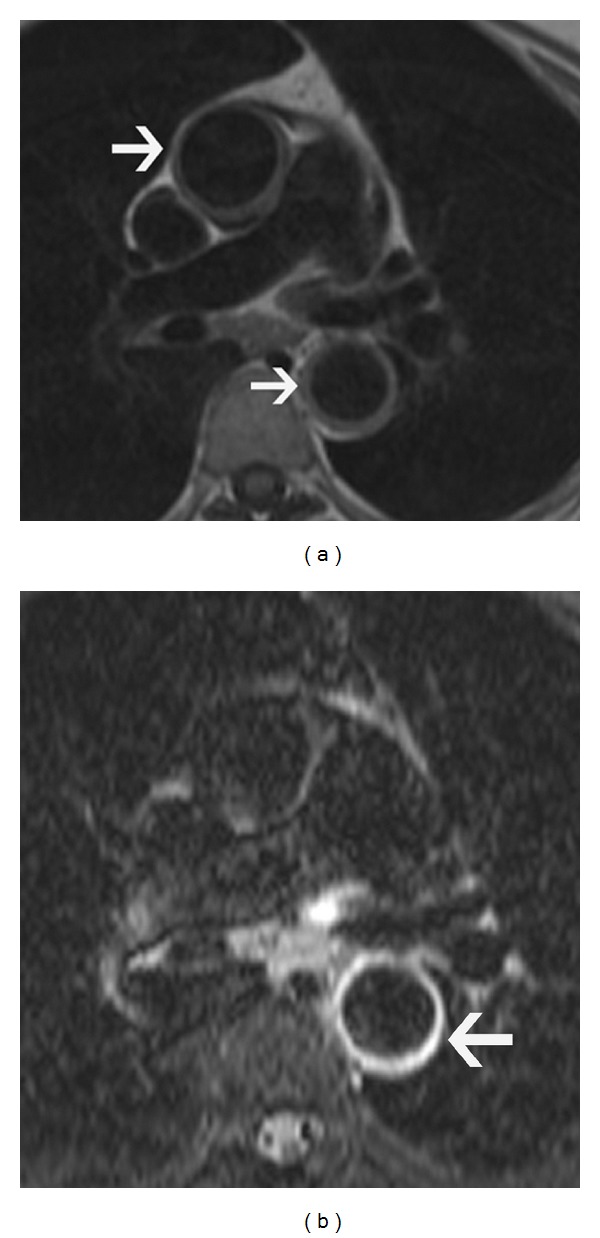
Giant  cell  arteritis—(a) axial T2-weighted double inversion recovery black blood image in a 68-year-old female patient with giant cell arteritis shows mild circumferential thickening of the ascending and descending aorta (arrow). (b) T2-weighted triple inversion recovery black blood image in the same patient with giant cell arteritis shows very minimal high signal of the descending aorta (arrow), which is consistent with mild active arteritis.
